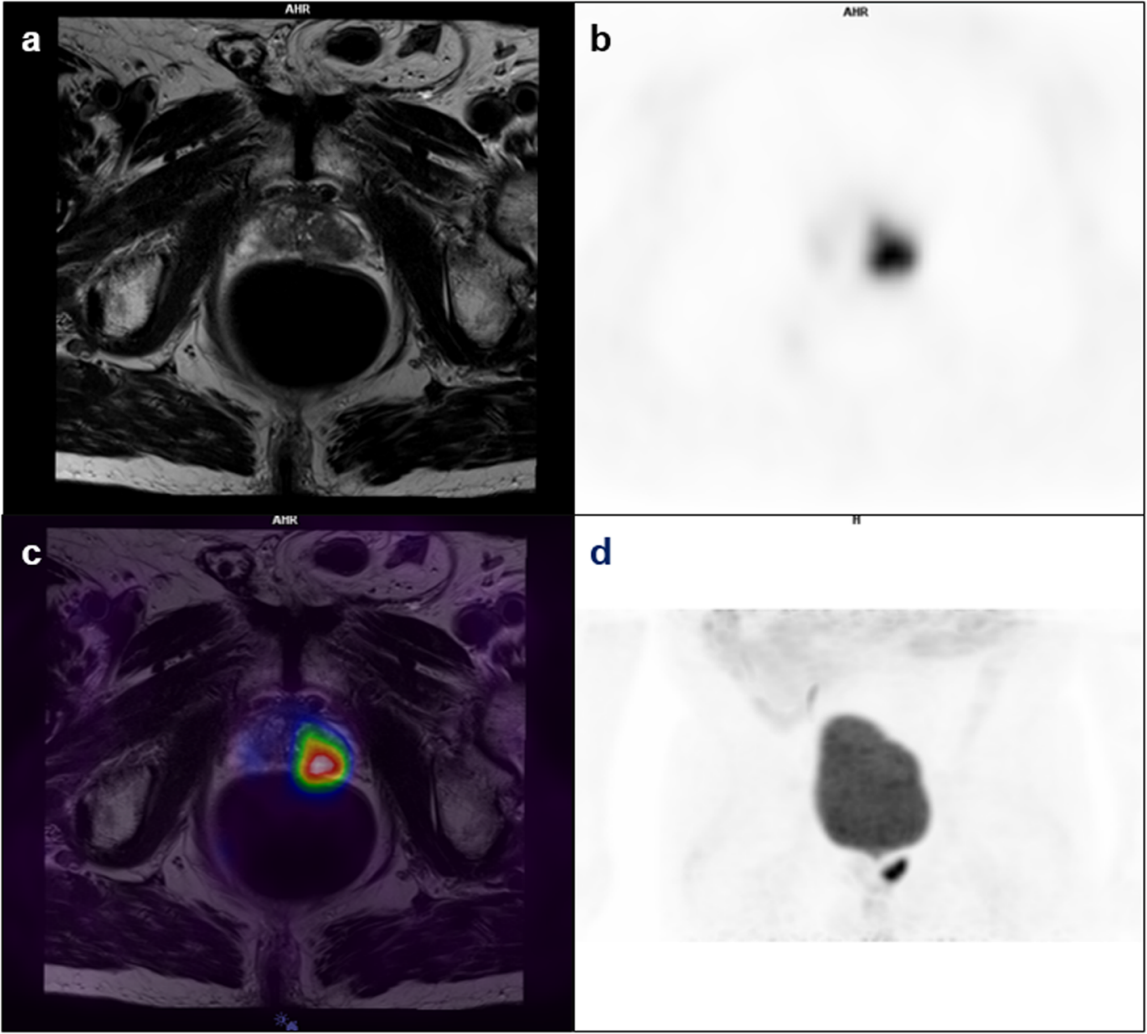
Primary prostate cancer patient (PSA 5.57 ng/mL) presenting an intense focal ^68^Ga-PSMA-11 uptake in the left lobe of the prostate (SUV 45–60 min: 13.27). MR T2-weighted image (a), averaged dynamic PET 45–60 min post-injection (b), PET/MR fused image (c), and whole-body maximum intensity projection of the pelvis (d)
